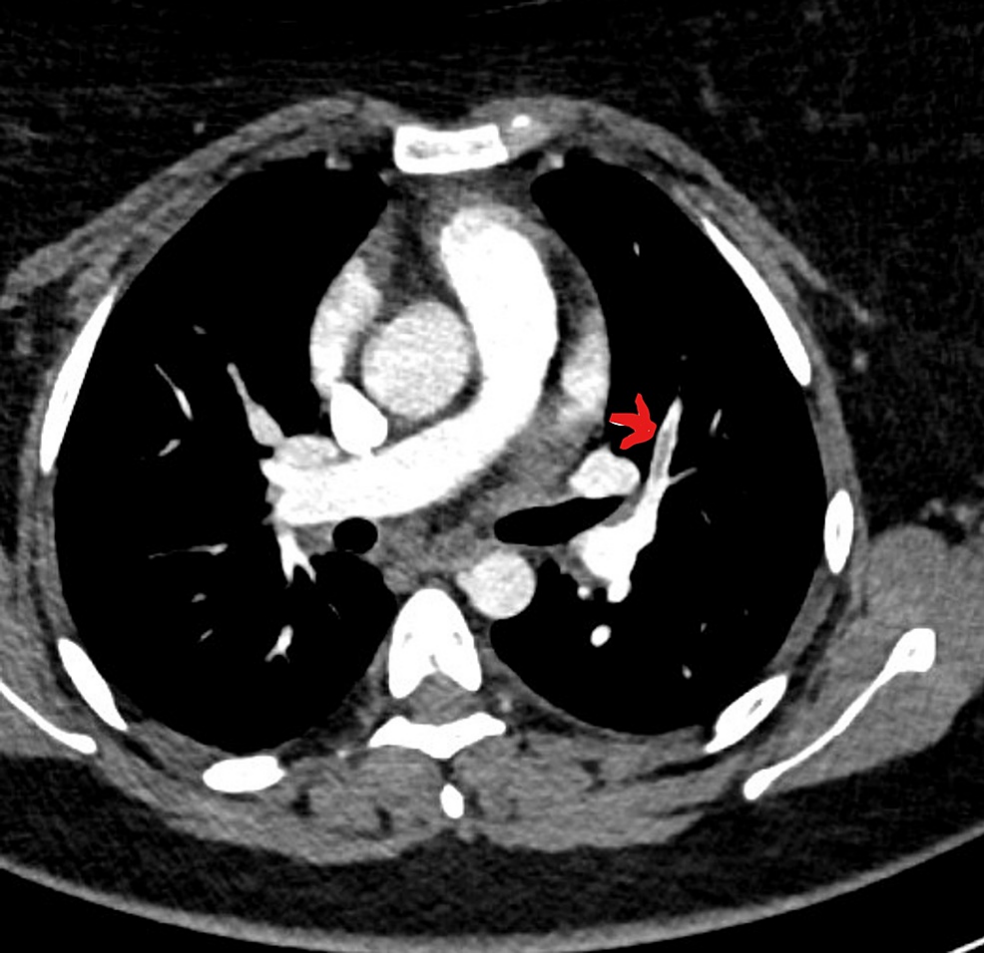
CT pulmonary angiogram (CTPA) showing segmental pulmonary embolism
